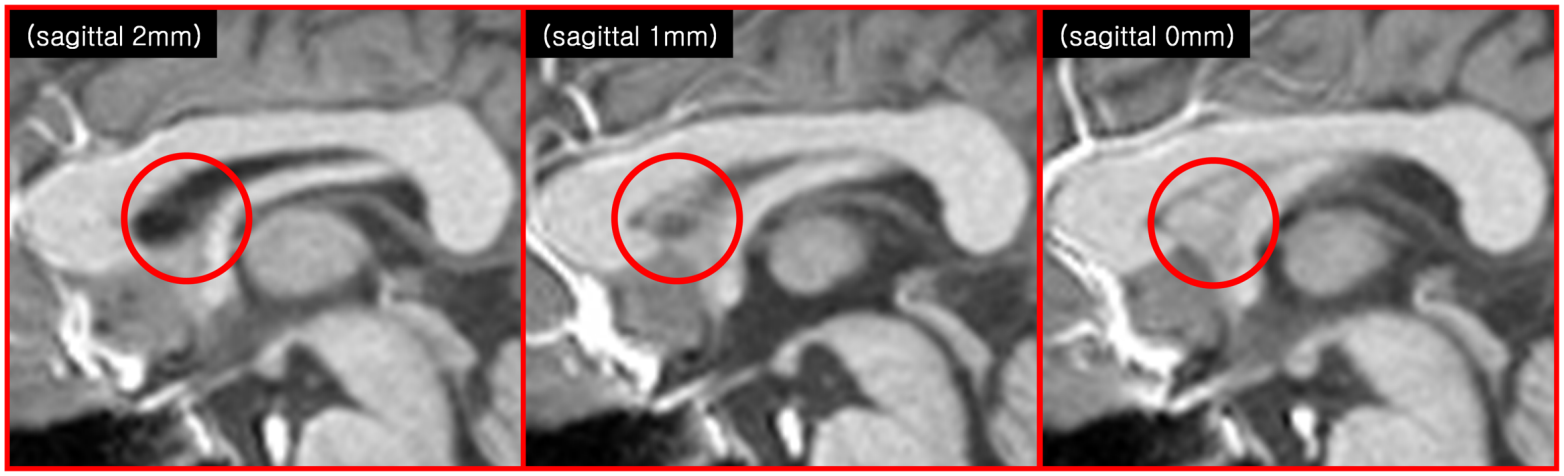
Another indication of the SPT is seen in the anatomical images of modern MRI scanners (3T T1-weighted sagittal MRI images). The images at 1 and 0 mm show some faint structures in the septum pellucidum area (red circle), but not in the image at 2 mm.
